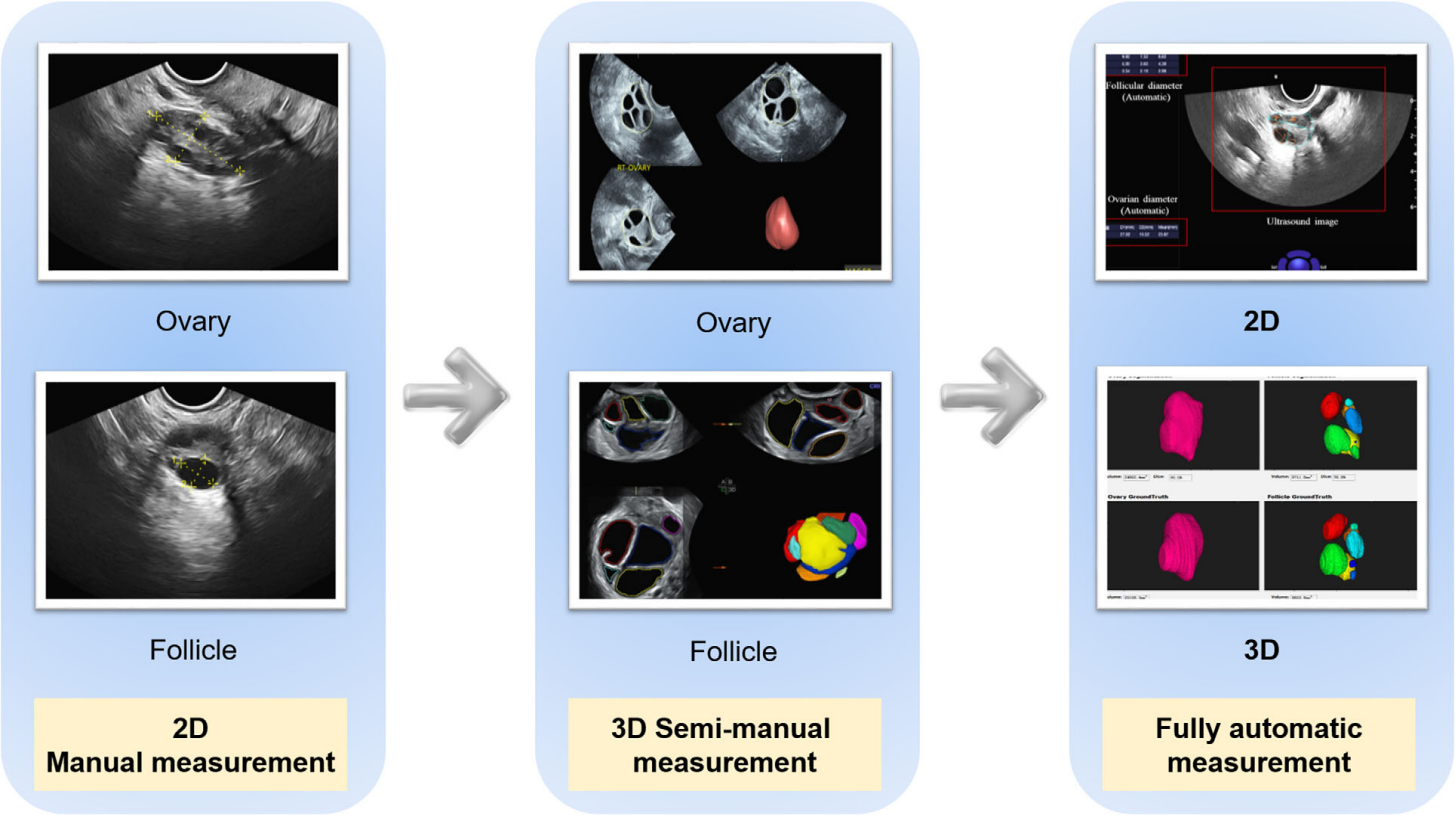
Advanced ultrasound techniques in follicular monitoring.
